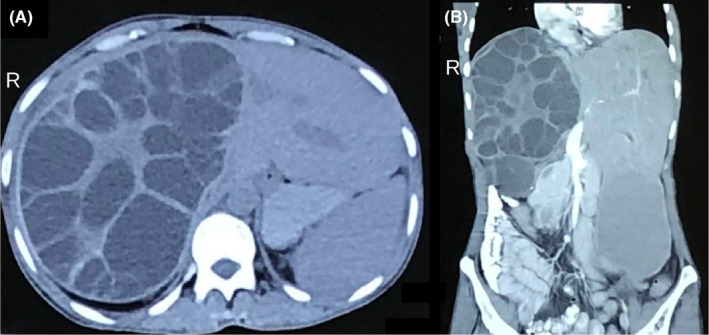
Axial (A) and coronal view (B) of computed tomography scan of the abdomen showing enlarged liver and cystic lesion with internal septations in liver.
